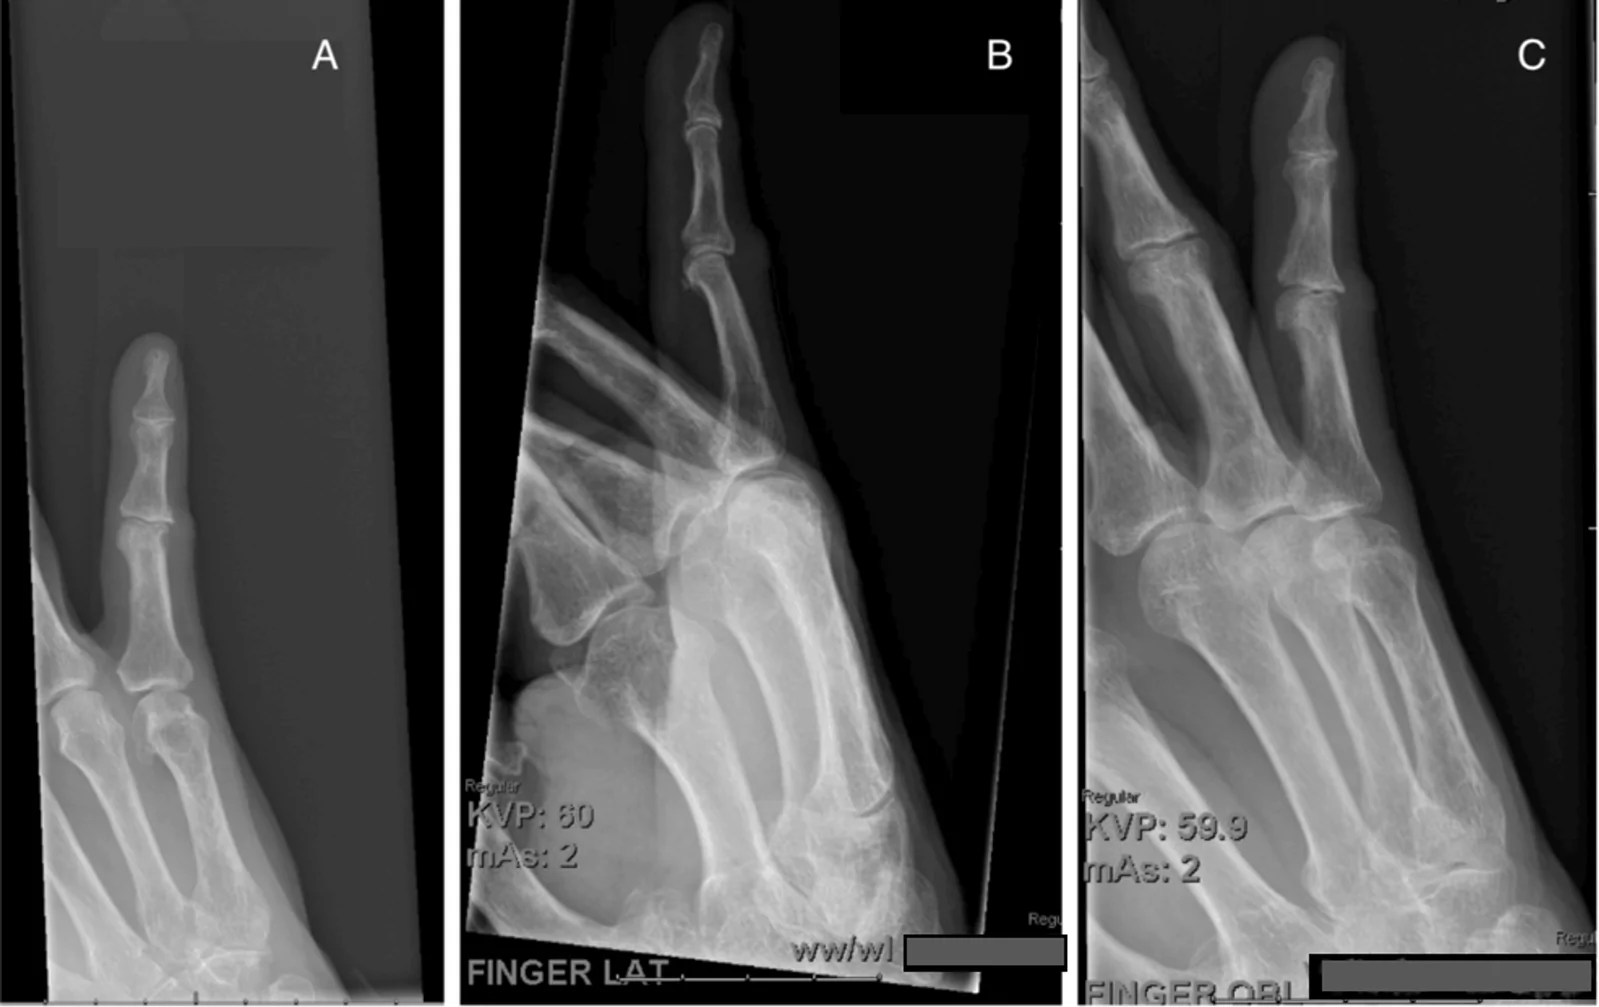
Post-reduction anteroposterior (A), lateral (B), and oblique (C) X-rays showing a 2-mm fracture fragment volar to the head of the right fifth proximal phalanx, with an apparent donor site at the base of the volar middle phalanx.
